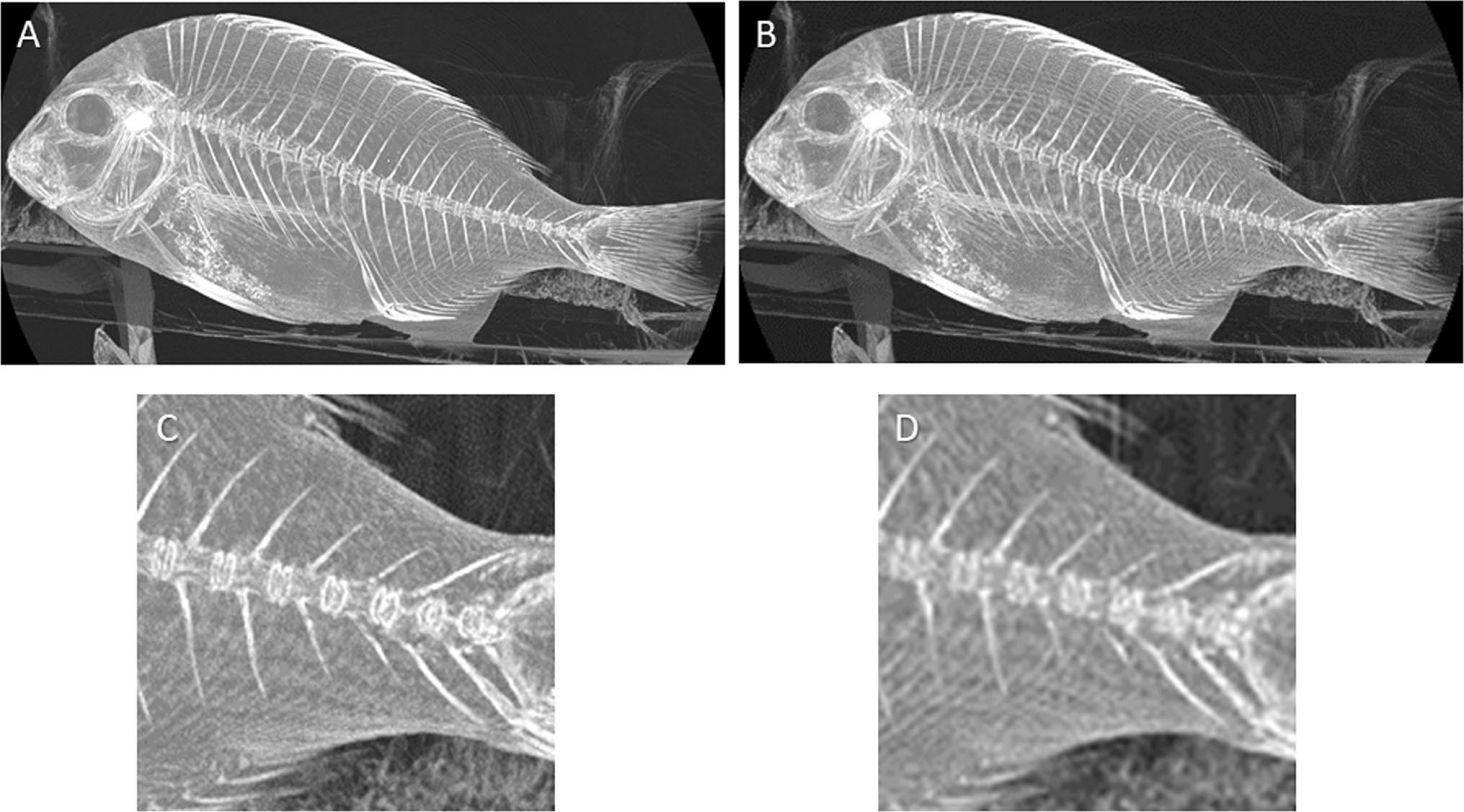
Maximum intensity projection images of fish (unpublished our own data). A PCD CT image in high resolution mode (matrix: 1024 × 1024, slab thickness: 45.75 mm). B PCD CT image with low resolution equivalent to conventional EID CT (matrix: 512 × 512, slab thickness: 45.75 mm). C Magnification of (A). D Magnification of (B). The overall structure is sharply delineated in (A and C) compared with (B and D)
